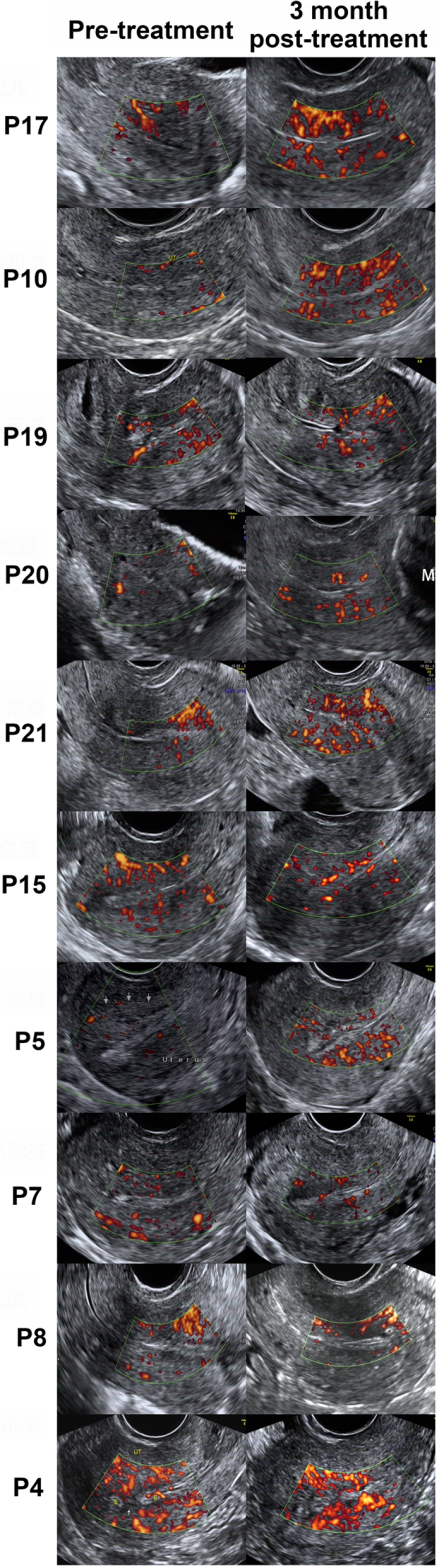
Blood flow of ten patients before and after UC-MSC/collagen treatment by Doppler ultrasound
